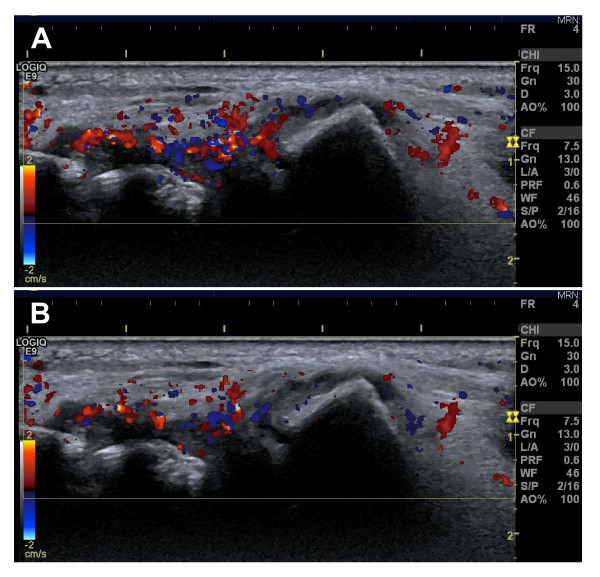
Image of the ulnar position of the wrist in a patient with rheumatoid arthritis. A: image with maximal Doppler activity. B: image with minimal Doppler activity. The two images are from the same cine-loop and are only a few seconds apart.
